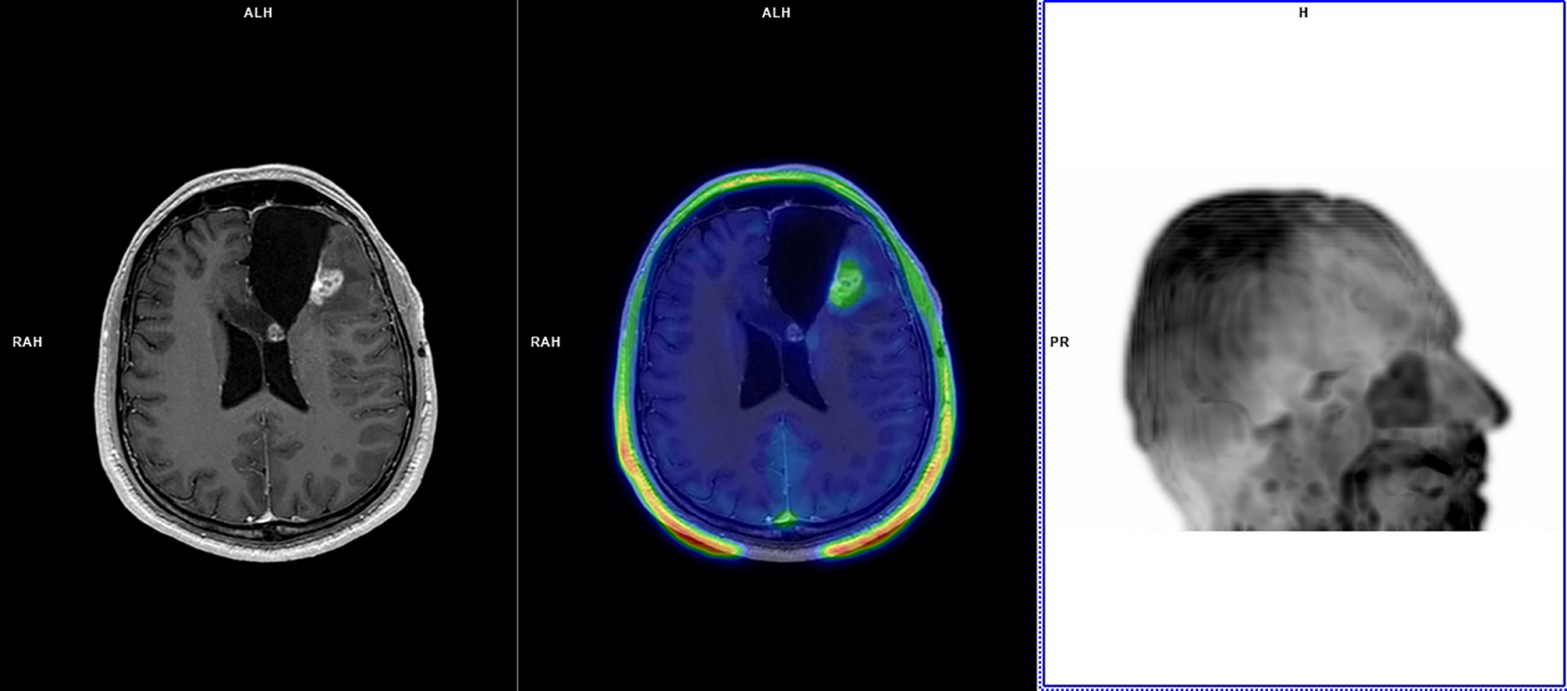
A 35-year-old patient with left frontal glioblastoma (IDH wild-type; MGMT methylated) treated with partial resection in August 2017, chemoradiation (completed in November 2017), and four cycles of adjuvant temozolomide (last cycle in March 2018). In August 2018, left frontal cavity re-irradiation was performed for glioblastoma relapse. Follow-up MRI scans in November 2018 demonstrated an increasing pseudonodular area of contrast-enhancement along the left frontal surgical cavity without functional indices abnormalities, deemed dubious for GR. ^18^F-FET PET performed in December 2018 demonstrated faint tracer uptake at late static imaging (TBRmax: 1.6) and TAC pattern 3 with TTP at 35 min. ^18^F-FET PET was considered suggestive of TICs. MRI demonstrated disease progression in August 2019 (PFS: 8 months; OS not reached), and bevacizumab was commenced. At last MRI follow-up (January 2021) MRI demonstrated stable disease.
